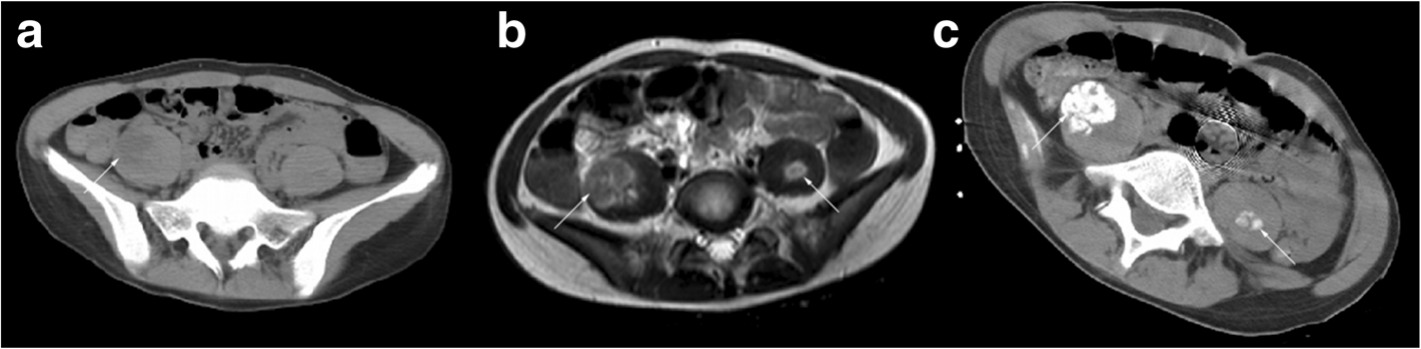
The pelvic CT and MRI of the patient. a The 4th day on admission, pelvic CT revealed hypointensity and edema of the right psoas major (*arrow*). b The 10th day on admission, T2 weighted MRI showed abnormal signals of the bilateral psoas major (*arrows*). c The 17th day on admission, pelvic CT showed diffused ossifications of the bilateral psoas major (*arrows*)
